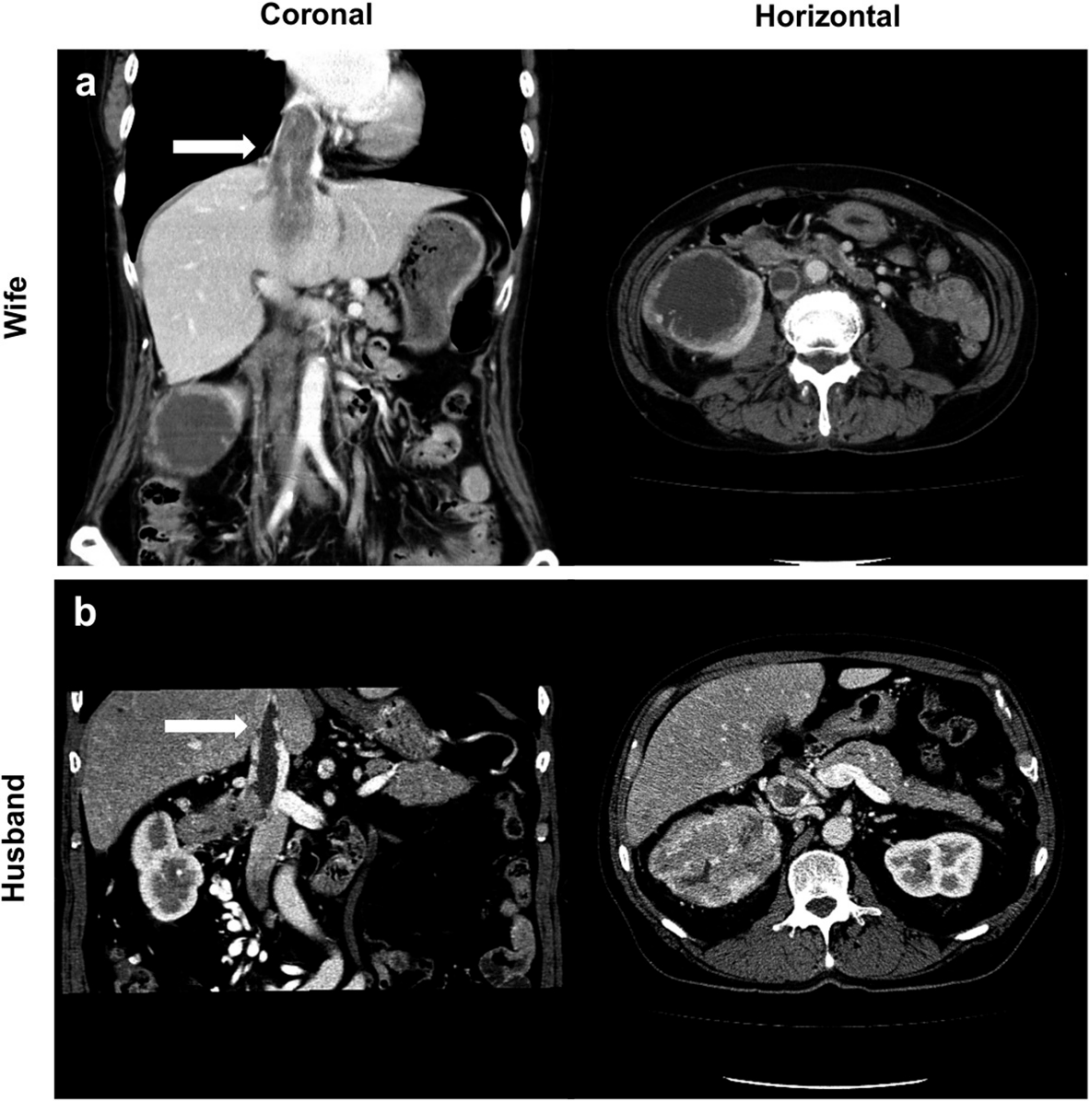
Right renal tumor and tumor thrombus in a married couple. (a) Case 1: Abdominal CT revealed that the tumor thrombus extended into the right atrium. (b) Case 2 (the husband of Case 1): The tumor thrombus extended into the intrahepatic inferior vena cava.
